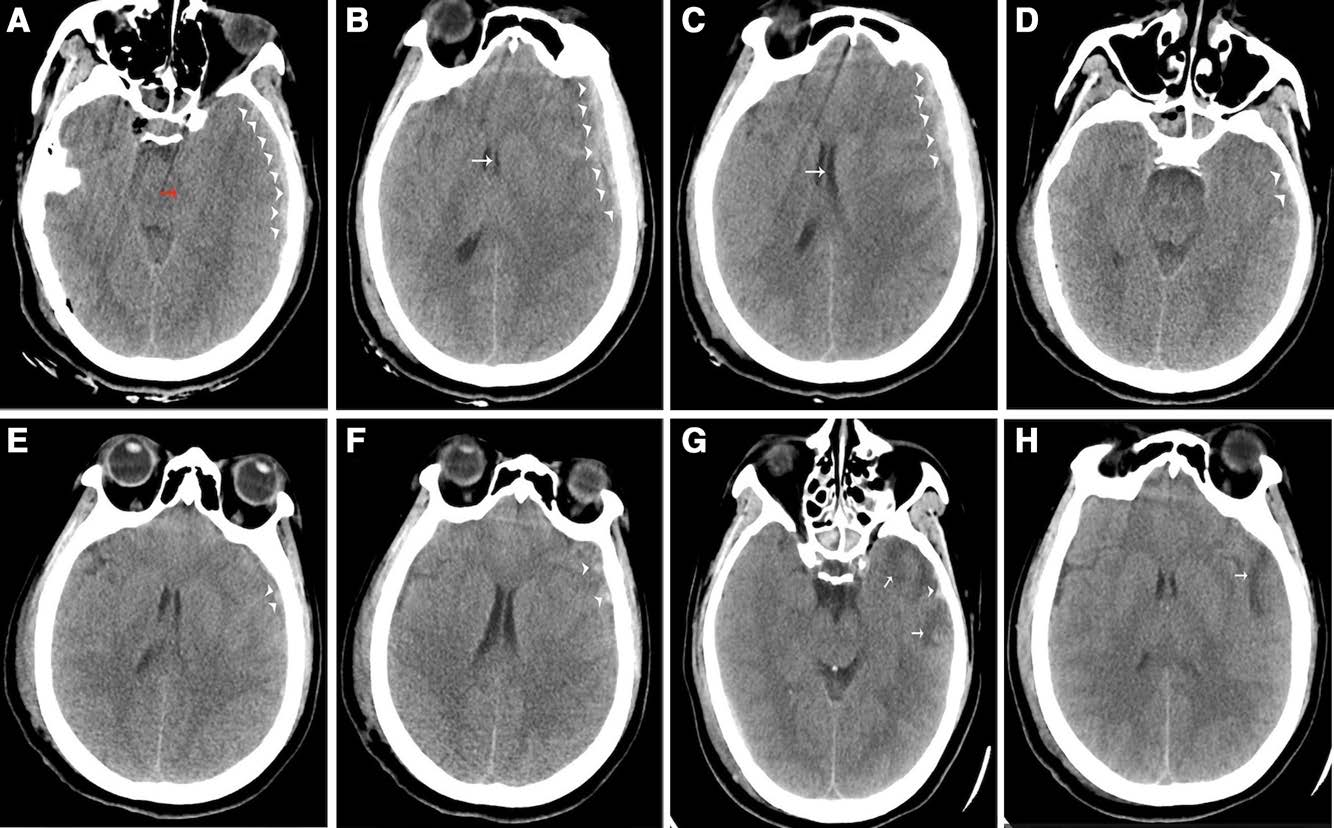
CT images taken during admission, 4 hours after admission, and 72 hours after admission. (A–C) CT 1 hour after trauma revealed left temporal and frontal subdural hematoma (arrowheads), brainstem compression (red arrow), and midline shift (white arrows). (D–F) CT 4 hours later showed disappearing subdural hematoma (arrowheads), healthy ambient cistern, and restored midline. (G and H) CT 72 hours later showed the barely visible subdural hematoma (arrowhead) and slight edema (white arrows).
